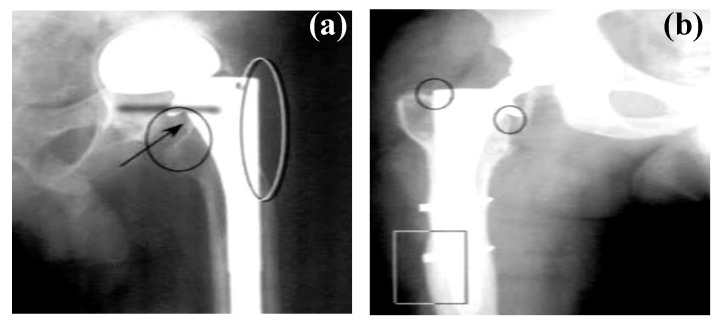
Radiographs of hip implants showing changes in bone mass due to stress shielding around an implant: (a) bone loss at the proximal region (indicated in the circled regions at the edge of the prosthesis) and (b) bone deposition (indicated by the rectangle) in the distal region of the implant . Obtained from [23], with permission from Wiley online library, 2018.
